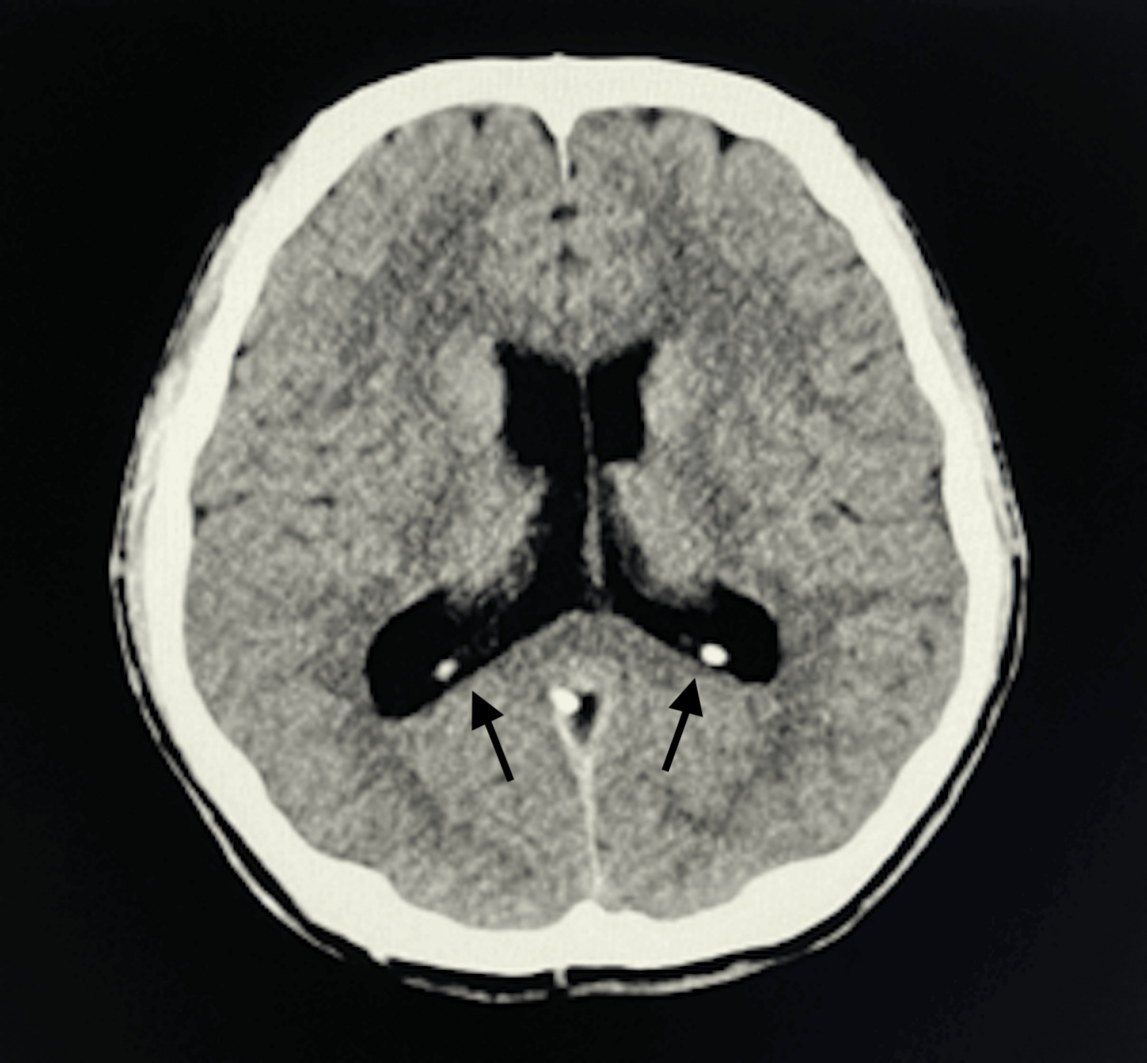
Brain computed tomography. Axial CT demonstrating marked enlargement of the posterior bodies and trigones of the bilateral lateral ventricles (arrows).
